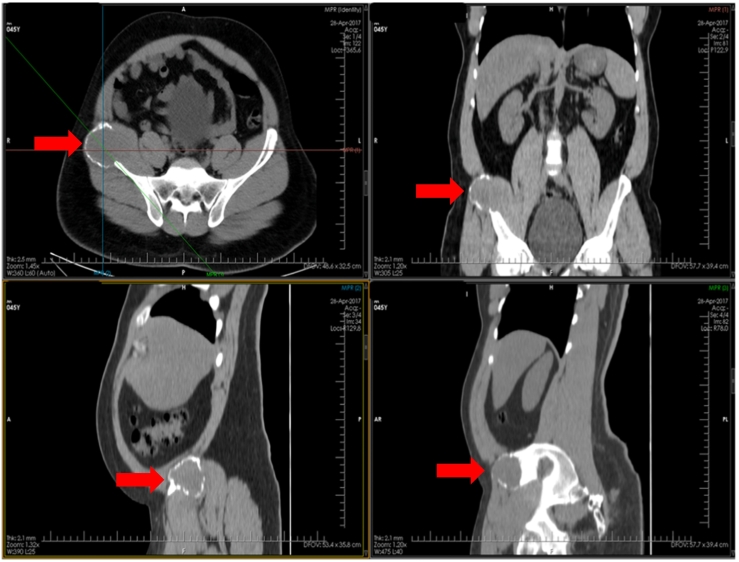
Initial CT scan of abdomen (axial, coronal and sagittal view) – Reformatted images of non contrasted CT abdomen showing an expansile hypodense mass involving the right iliac bone (red arrow).
